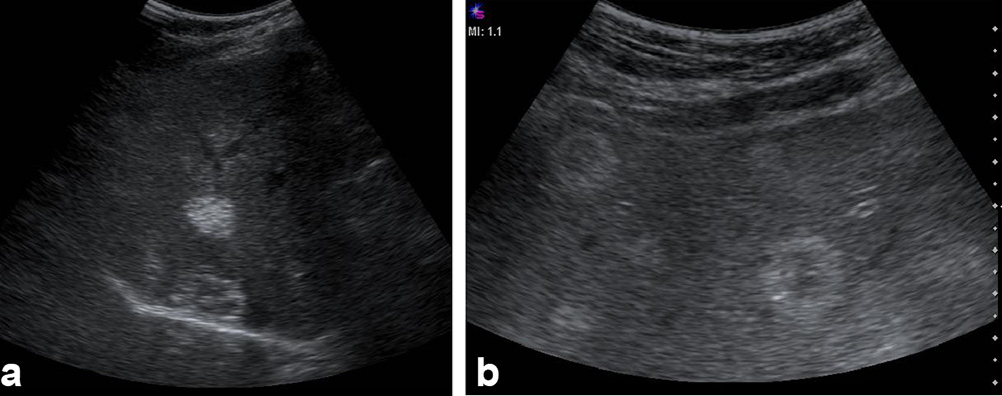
Liver Ultrasound: (a and b) Multiple heterogeneous, predominantly echogenic hepatic lesions were identified, distributed among all liver segments. Some had target-like or annular appearance and others had calcifications. Some had a pattern similar to “hailstorm” described in echinococcosis.
